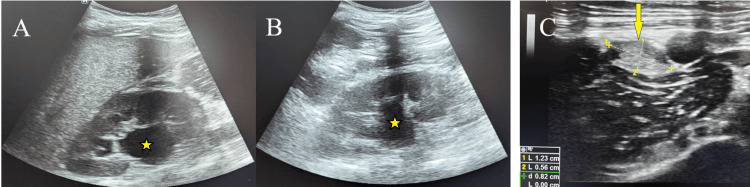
Case 1: abdominal and pelvic ultrasound Abdominal ultrasound showing dilated pelvicalyceal system (yellow star); A: right kidney; B: left kidney; C: ectopic testis in the right inguinal canal (yellow arrow).
